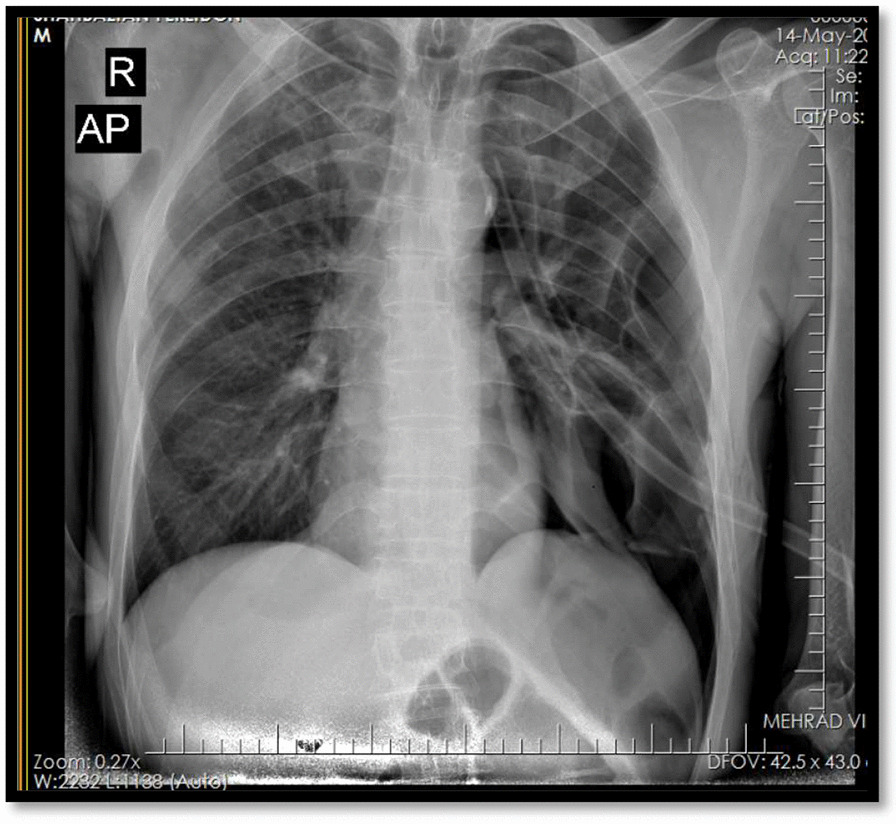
Chest radiography (2nd admission), not-resolved left sided pneumothorax after the chest tube insertion
